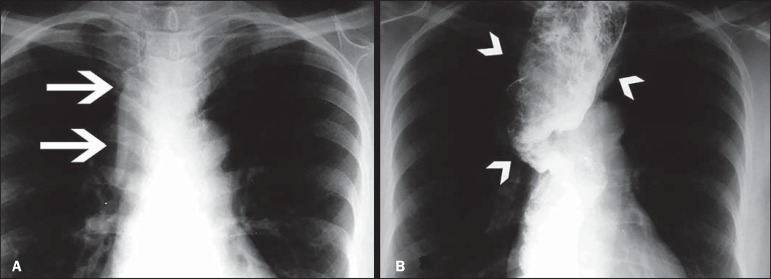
Grade IV megaesophagus. A: Posteroanterior X-ray showing
